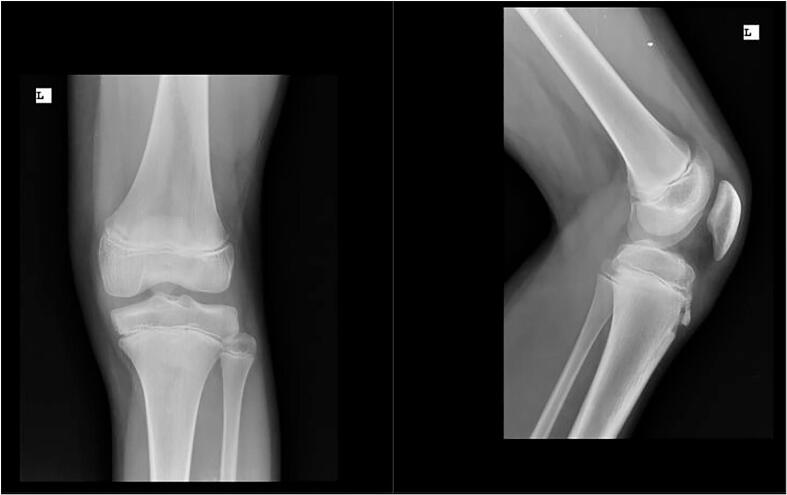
The X-ray of the affected knee.
